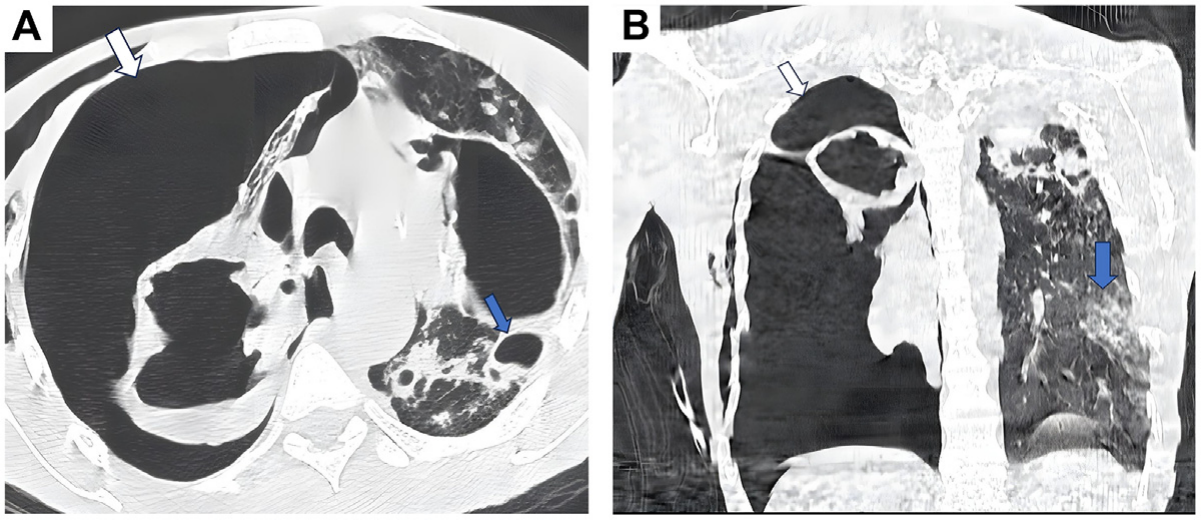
A. Axial chest CT scan (lung window) showing a large right-sided tension pneumothorax (white arrow) with collapse of the right lung. Note the presence of cystic and bronchiectatic changes with apical pseudo-cavitary lesions and areas of bullous emphysema, suggestive of chronic postinfectious sequelae (blue arrow). B. Coronal chest CT scan (lung window) showing a massive right-sided tension pneumothorax (white arrow) with near-total collapse of the right lung and leftward mediastinal shift. The left lung demonstrates fibro-bronchiectatic changes and parenchymal opacities consistent with postinfectious sequelae, likely secondary to prior pulmonary tuberculosis (Blue arrow).
